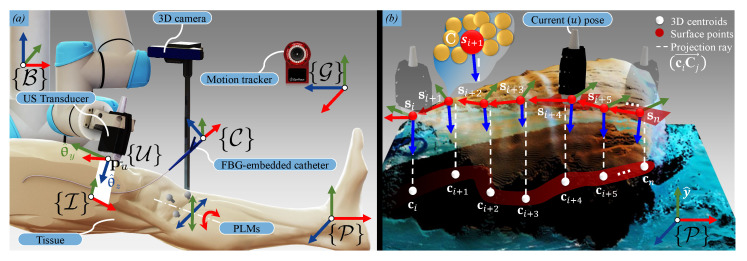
Intra-operative ultrasound (US) transducer pose planning. (a) In this study, we integrate three imaging modalities: a 3D depth camera, a US transducer, and a motion tracking system. Additionally, data from the Fiber Bragg Grating (FBG) sensors are fused with imaging data to provide real-time shape information of the endovascular catheter. This information is combined with the reconstructed environment from the pre-operative planning to visualize the catheter inside the arterial volume, which is subjected to Periodic Limb Movements (PLMs). All reference frames are indicated in brackets ({}). (b) Surface points ($s_{i}$) are obtained by vertically projecting the arterial centroids ($c_{i}$) obtained from Algorithm 1, and finding the closest point on the surface point-cloud dataset ($C_{j}$). A trajectory for the US transducer is then calculated along the tissue surface. A proportional-integral (PI) controller positions the transducer, while its orientation is controlled by a P-controller. Together, these controllers realize the pose of the transducer (obtained from Algorithm 2) at a 3D surface target point.
